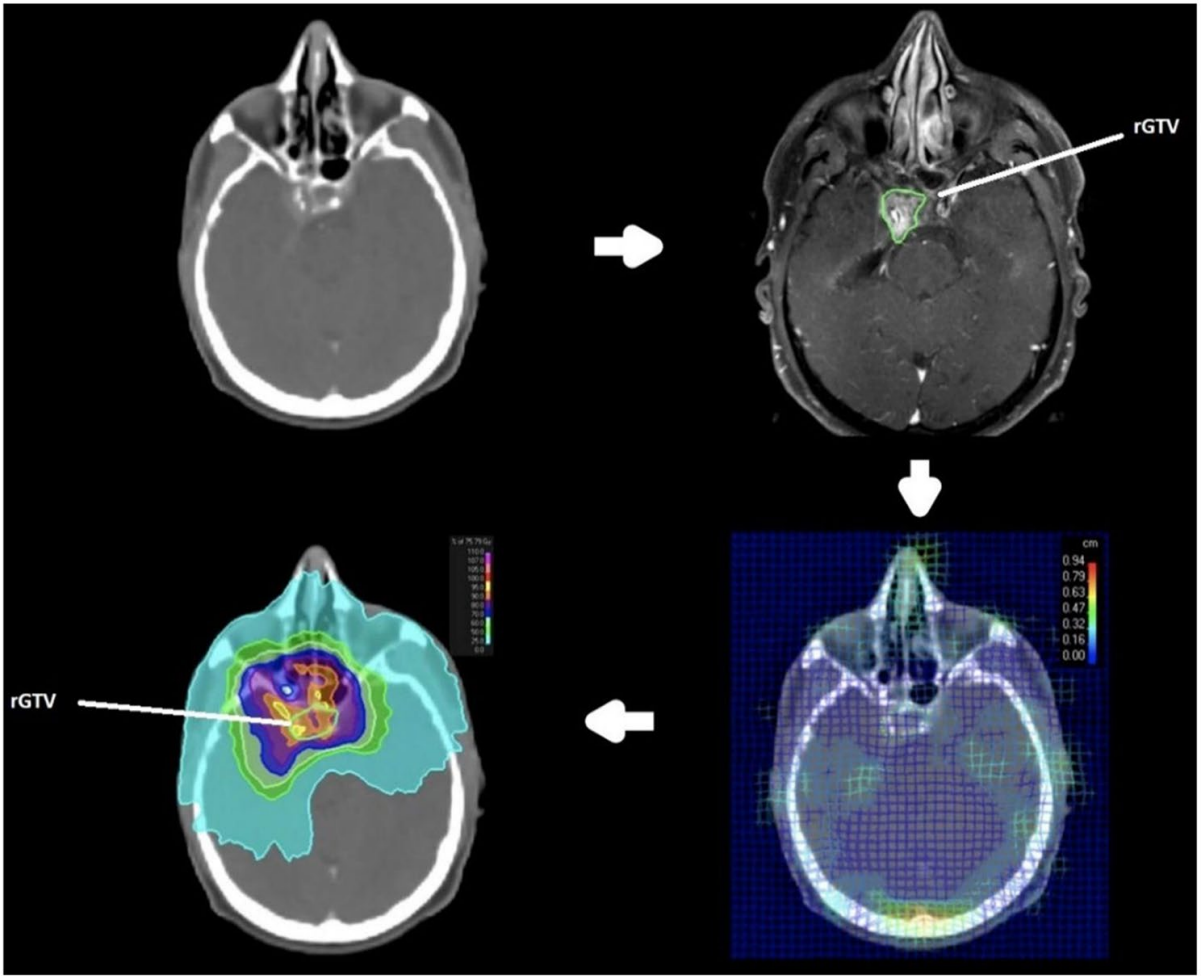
Process for defining recurrent gross tumor volume (rGTV) and applying deformable image registration (DIR). Top left: The initial planning CT (pCT) scan and radiotherapy treatment plan were accessed. Top right: A follow-up CT or MRI scan (rCT/rMRI) capturing tumor recurrence was obtained for rGTV delineation. Bottom right: The recurrence imaging (rCT/rMRI) was aligned with the original pCT scan. Bottom left: The identified rGTV from the rCT/rMRI was deformed onto the co-registered pCT
